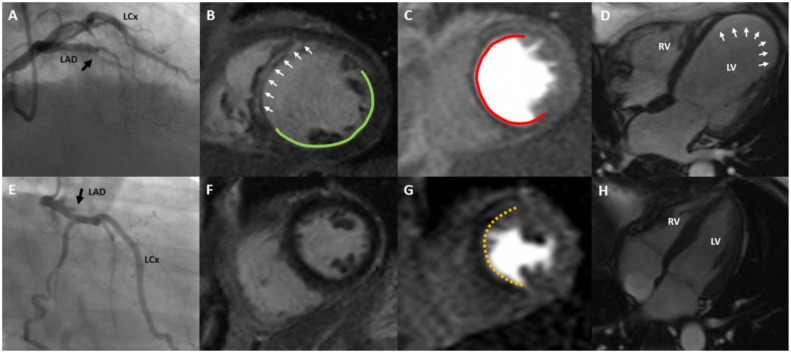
Two case examples of LAD CTO with CMR-based myocardial characterisation. (A) A coronary angiography image in the right anterior oblique (RAO) cranial view, showing a chronic total occlusion (CTO) in the left anterior descending (LAD) artery, marked by a black arrow. (B) A mid-left ventricular (LV) short-axis T1 mapping image highlighting a large myocardial scar occupying more than 50% of the wall thickness. The scarred region (LV septum) is indicated by white arrows, while the normal myocardium is outlined in green. (C) A single frame from a dynamic stress perfusion scan (short-axis, mid-ventricle) revealing a fixed perfusion defect across the LAD territory in the LV septum, outlined in red. (D) An apical four-chamber (A4C) cine view showing thinning of the LV apex indicated by white arrows. (E) A postero-anterior caudal angiographic view displaying a proximal LAD CTO, marked with a black arrow. (F) A mid-ventricular short-axis T1 map showing no evidence of scar in the LV myocardium. (G) A frame from a mid-ventricular short-axis stress perfusion study showing a stress-induced perfusion defect in the septal and anterior segments of the LV, marked by a yellow outline. (H) An A4C cine image of the LV, providing structural visualisation without additional findings noted.
